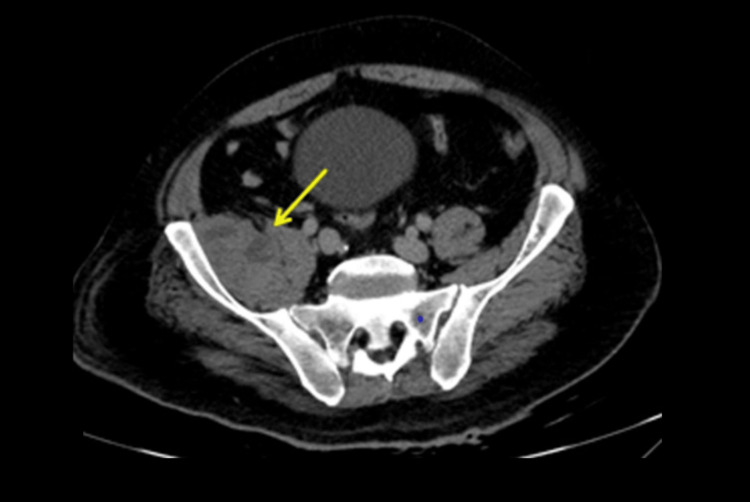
Contrast-enhanced abdominal computed tomography Axial contrast-enhanced CT demonstrating enlargement of the right iliopsoas muscle with a heterogeneous intramuscular collection (arrow) consistent with an iliopsoas hematoma.
